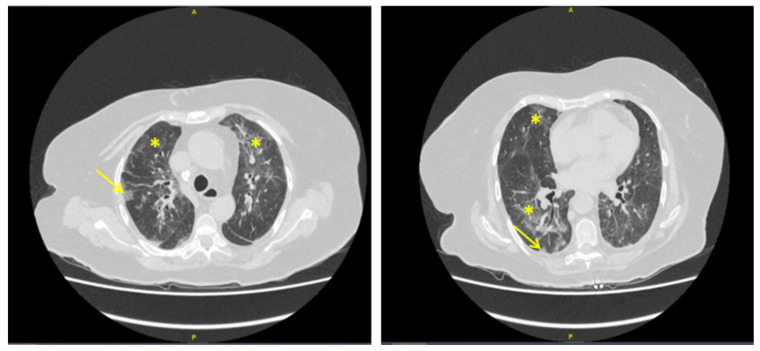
Chest computed tomography (CT) of an 82-year-old woman with advanced lung adenocarcinoma successfully treated with gefitinib and admitted for dyspnea two days previously. Bilateral ground glass opacities (*), and subpleural condensation (→) were interpreted as possible diffuse COVID-19 pneumonia. Note the absence of crazy paving aspect. RT-PCR on nasopharyngeal swab was negative for Sars-Cov-2 twice. A final diagnosis of Pneumocystis jirovecii pneumonia on gefitinib (a tyrosine kinase inhibitor) was retained after positive blood (1-3)-Beta-D-glucane and PCR for P. jirovecii on bronchoalveolar lavage.
